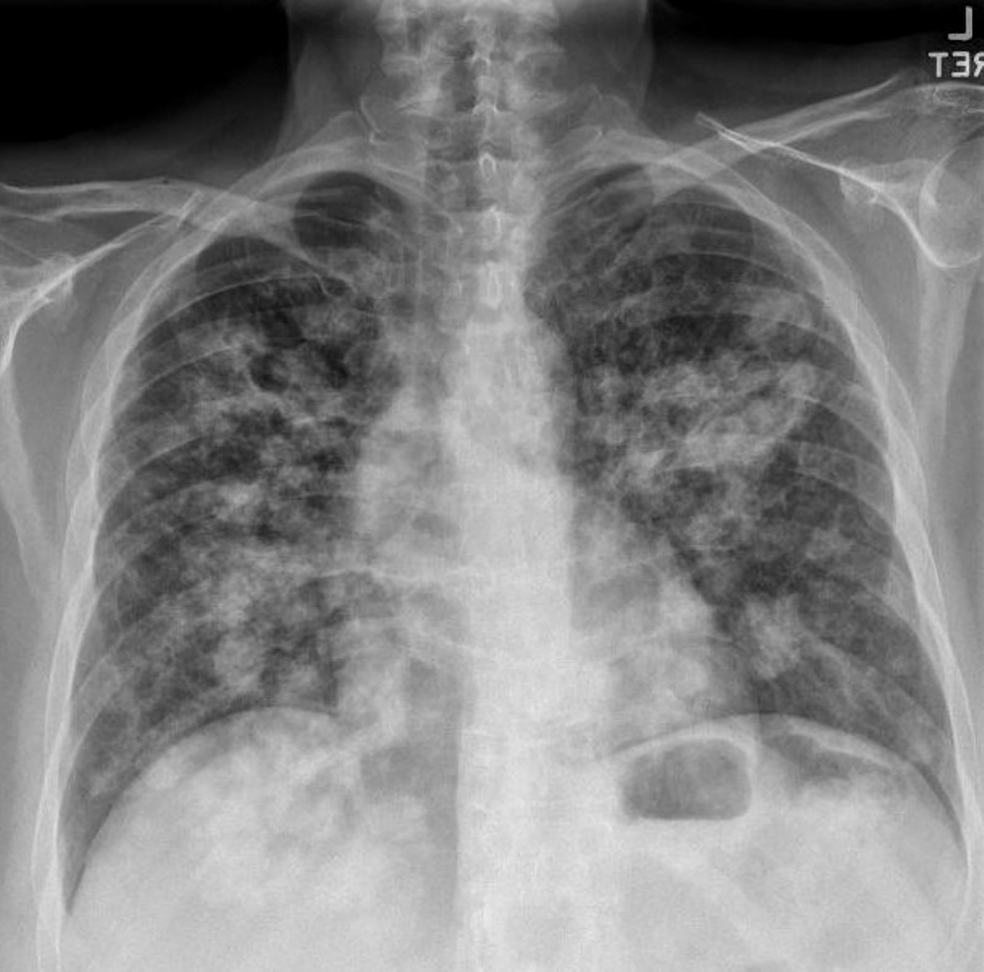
Chest X-ray showing bilateral nodular lung opacities concerning for metastatic disease
